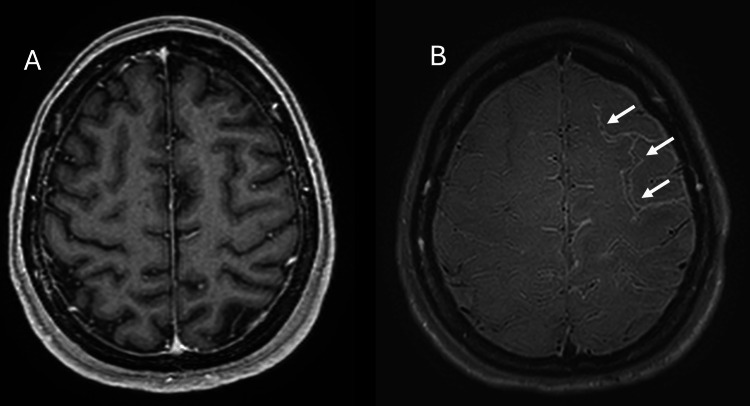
Axial MRI brain images in T1-weighted post-gadolinium (A) and vessel wall black-blood imaging (2B) sequences. Patchy leptomeningeal enhancement is observed in the left fronto-parietal region (arrow in B), with the vessel wall black-blood imaging sequence providing the best visualization.
